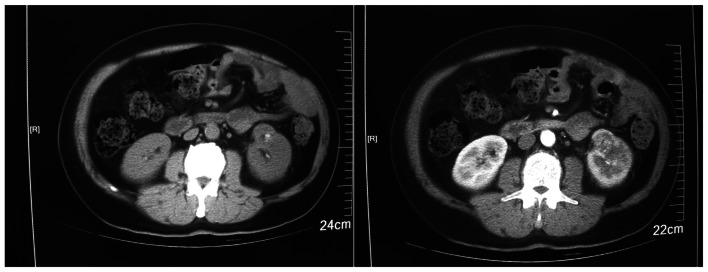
Abdominal computed tomography scan. A computed tomography scan revealed a renal mass with a mixed density in the lower pole of the left kidney measuring 5×4×5 cm.
